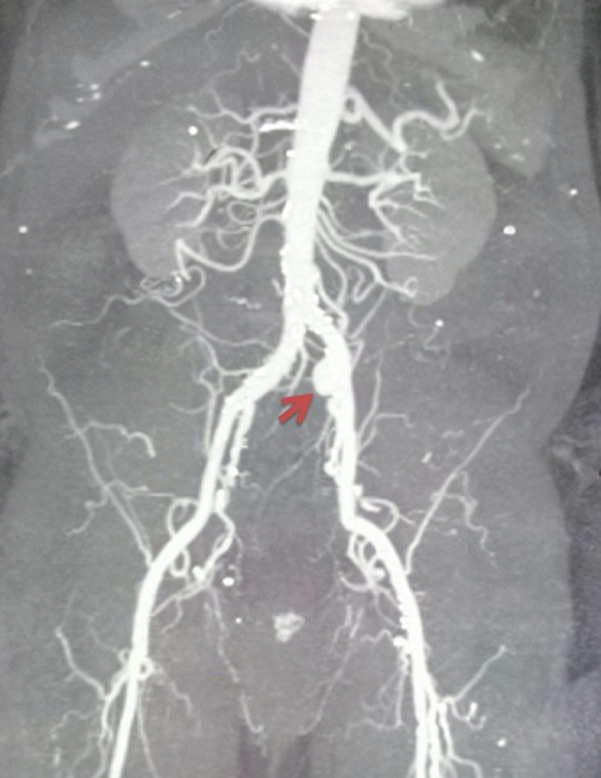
computerized tomography (CT) scan showing an isolated aneurysm of the left common iliac artery
